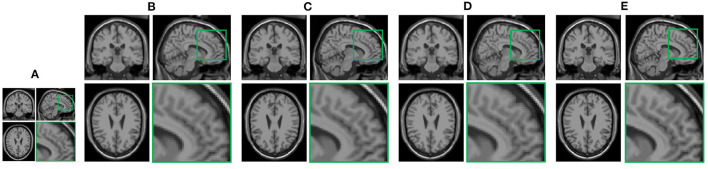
Reconstruction results and their corresponding details of synthetic brain MRI data from Brainweb database: (A) Low-resolution (LR) band with downsampling factor 2, (B) nearest neighbor (NN), (C) low-rank and total variation regularizations (LRTV), (D) TF-SISR, (E) nonconvex nonlocal Tucker decomposition with weighted 3D total variation (NNTDTV).
